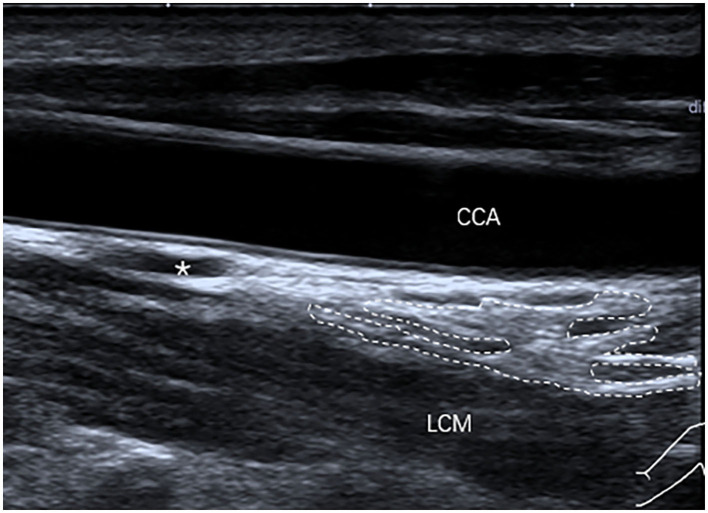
The SG, with a spindle shape (outlined) and hyperechogenicity, was located between the common carotid artery (CCA) and the longus colli muscle (LCM) and was under the inferior thyroid artery (*) on the longitudinal ultrasound image.
